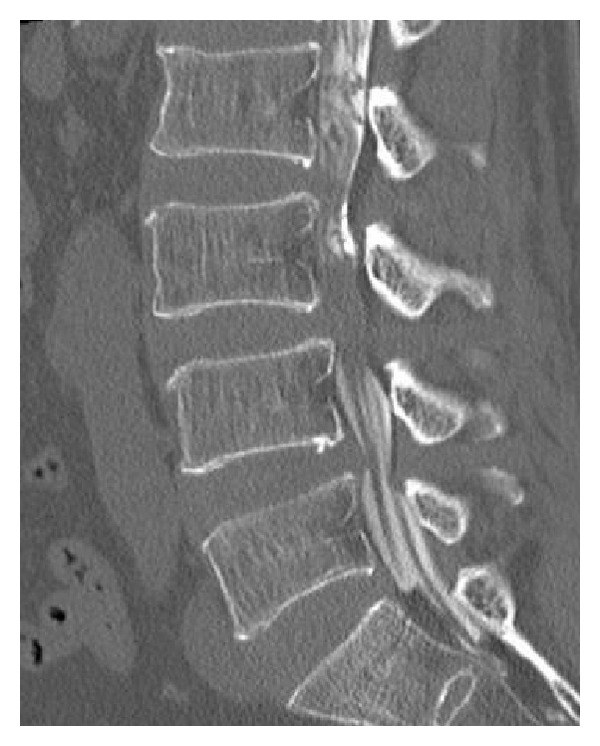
Preoperative imaging. Sagittal reconstruction of a postmyelography computed tomography (CT) scan demonstrates L2-5 multiple spinal stenosis and concurrent grade I degenerative spondylolisthesis at L3/4 and L4/5.
